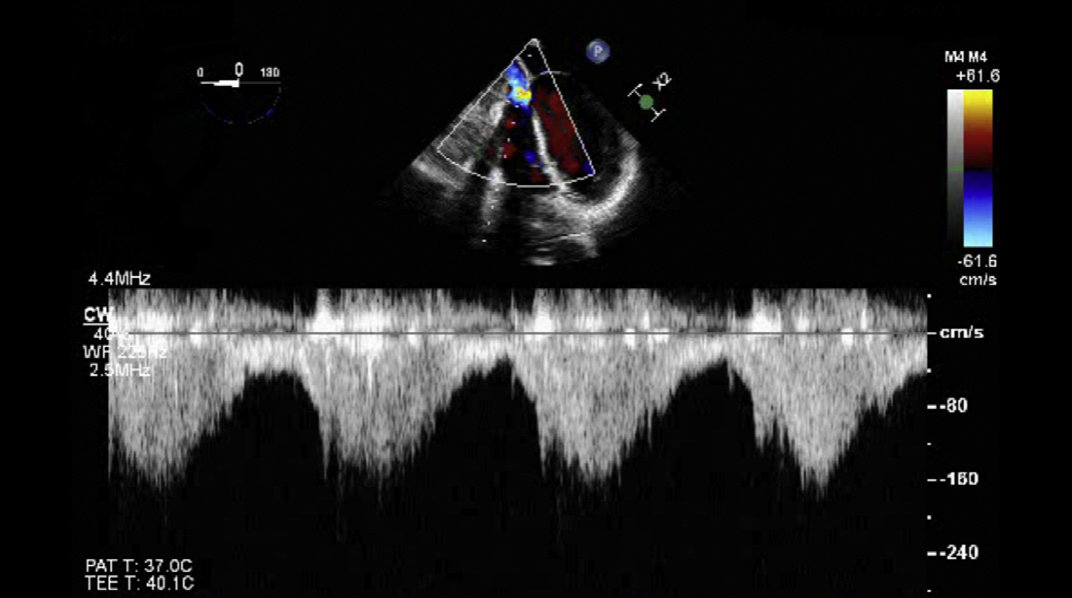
Transesophageal Echocardiography Showing no Functional Tricuspid Valve Stenosis
